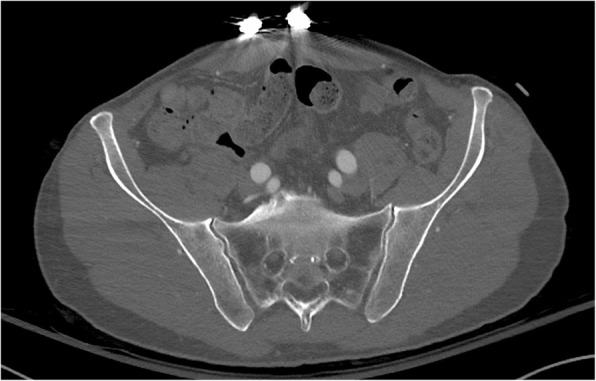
Computed tomography with binder *in situ* showing normal sacroiliac joints
